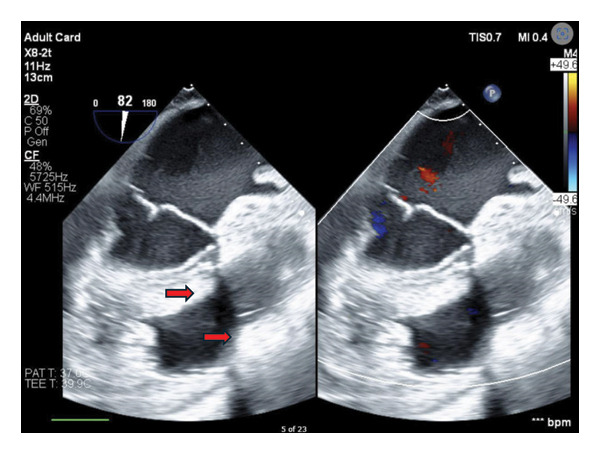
View of the RVOT on TEE demonstrating relief of subvalvular RVOT muscle bands (marked with red arrows) and resolution of flow acceleration on color Doppler.
